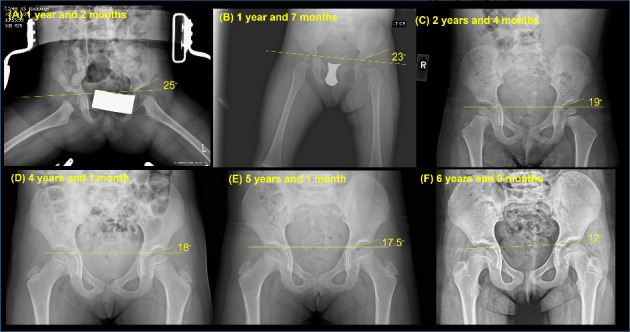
X-rays of the hip joints taken at (A) 1 year and 2 months, (B) 1 year and 7 months, (C) 2 years and 4 months, (D) 4 years and 1 month, (E) 5 years and 1 month, and (F) 6 years and 9 months.
